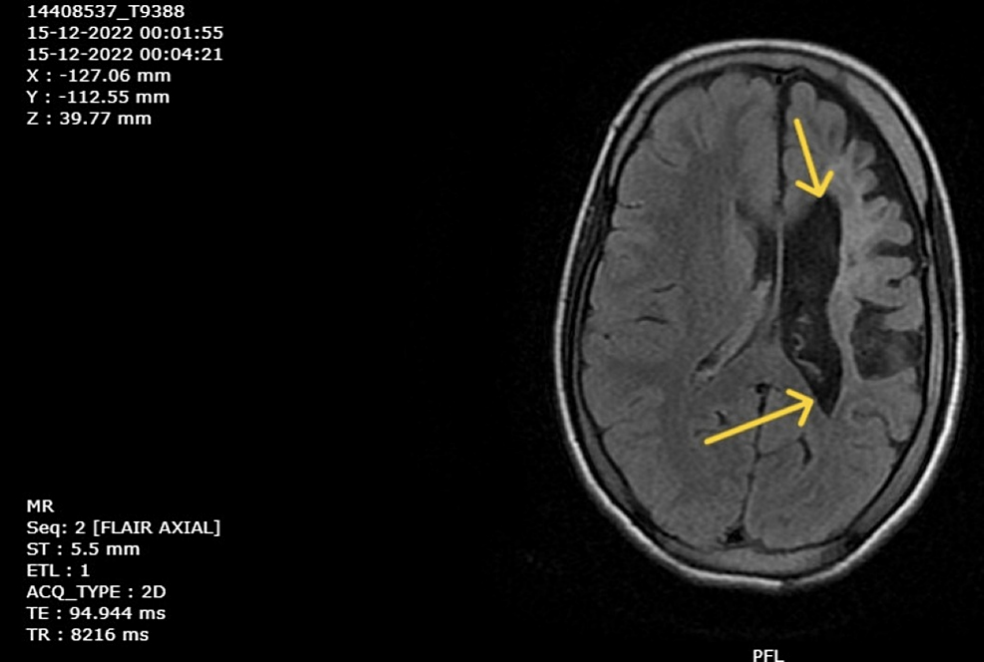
MRI of brain showing dilation of left lateral ventricle (December 10, 2022).
